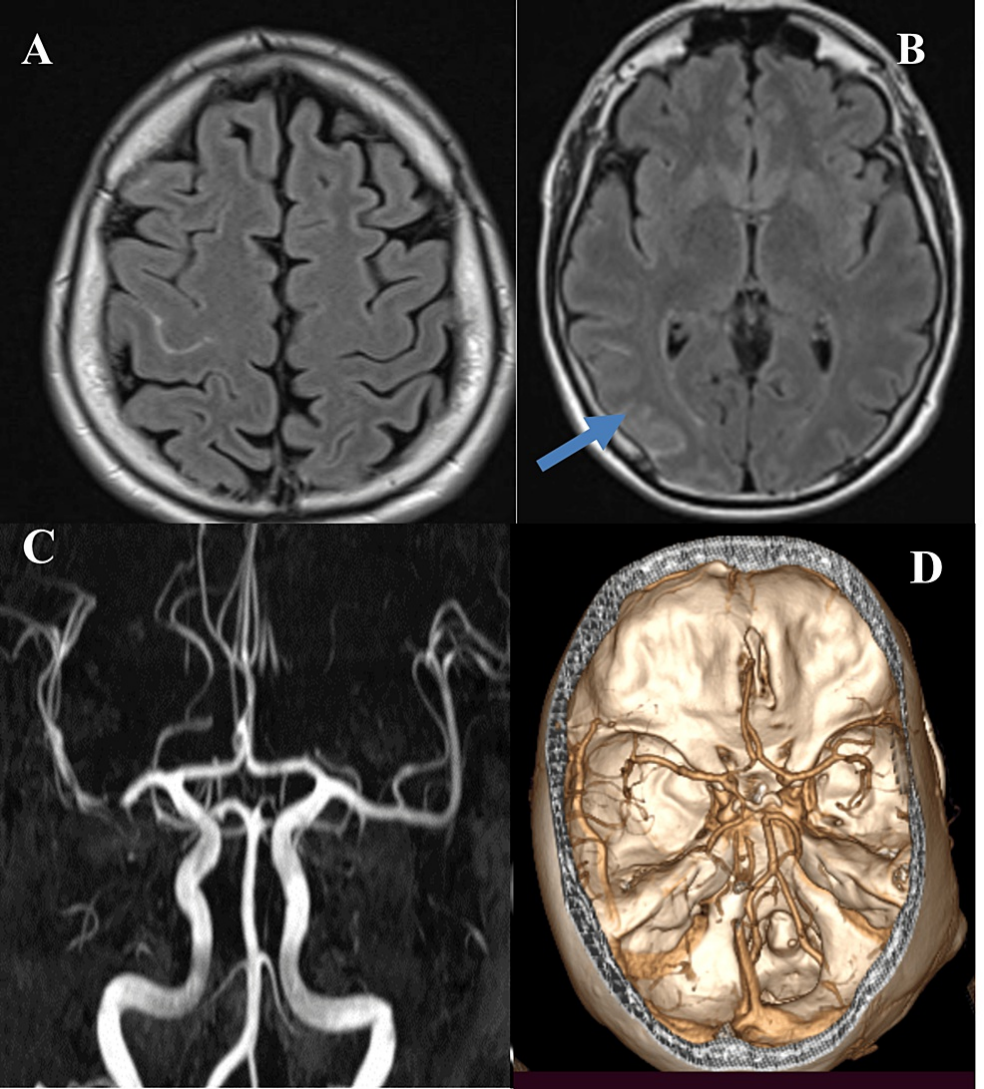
A 48-year-old man with sudden onset of left-sided incoordination and left-sided facial numbness intermittently over two weeks. The initial non-contrast CT (not shown) revealed focal SAH in the right central sulcus. The CT angiogram (not shown) was initially normal. (A-B) FLAIR MRI shows focal SAH in the right central sulcus and the parietooccipital region (blue arrow). (C) MRI angiogram demonstrated focal stenosis in the right middle cerebral artery. (D) Follow-up 3D CT angiogram after six months shows resolution of the right middle cerebral artery stenosis. CT: computed tomography; SAH: subarachnoid hemorrhage; FLAIR: fluid-attenuated inversion recovery; MRI: magnetic resonance imaging
